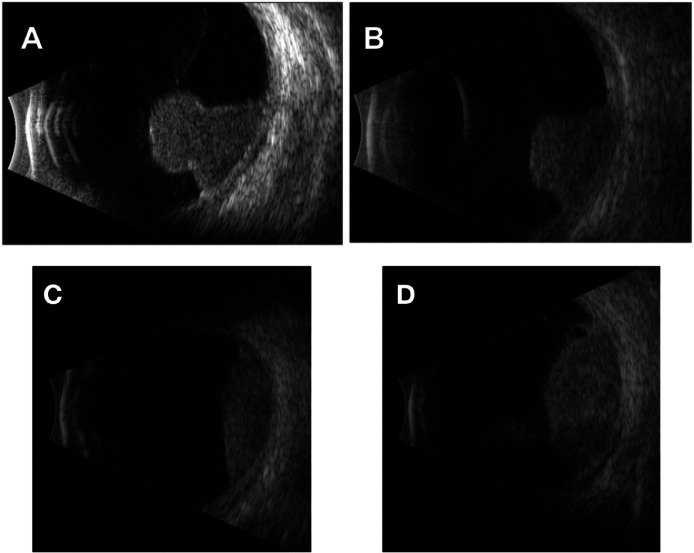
(A, B) Patient with local tumor control. (A) B-scan ultrasound of uveal melanoma before Gamma Knife stereotactic radiosurgery (GK SRS). (B) B-scan ultrasound of uveal melanoma post-GK SRS. (C, D) Patient with local tumor control failure. (C) B-scan ultrasound of uveal melanoma pre-GK SRS. (D) B-scan ultrasound of uveal melanoma post-GK SRS.
